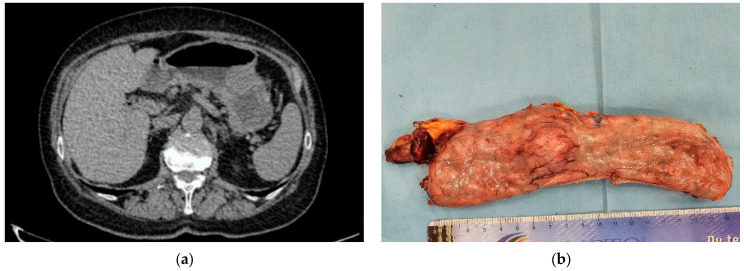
(a) CT aspect of gastric GIST. (b) Wedge resection for an endophytic gastric GIST.
